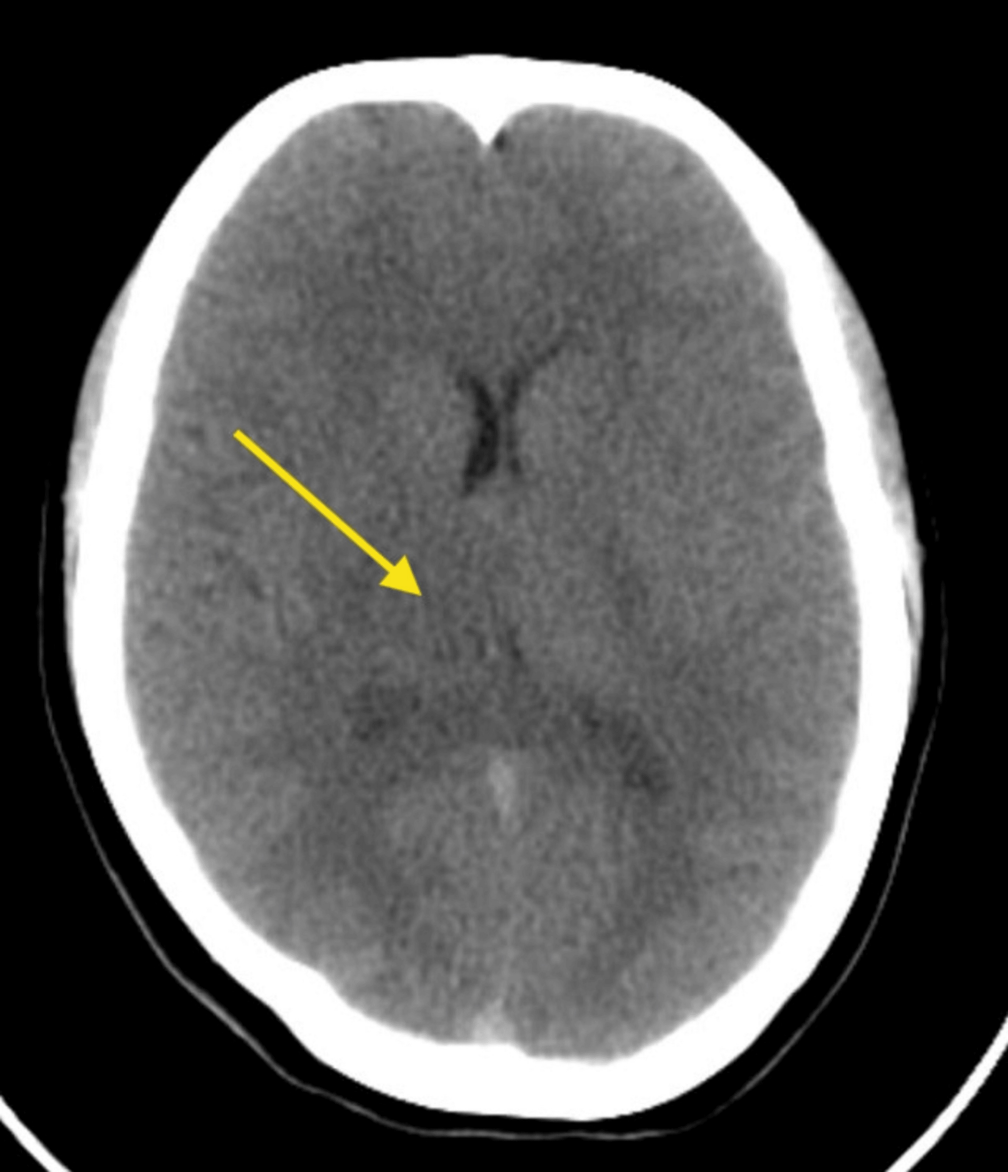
CT brain without contrast A hypoattenuating area involving the right thalamus (yellow arrow)
